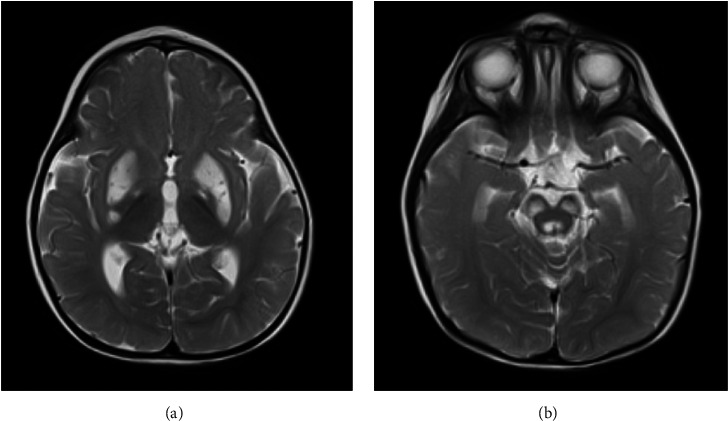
Brain MRI in the patient at the age of 51 weeks. (a) Axial, T2-weighted images showing bilateral high signal intensity in the lentiform nuclei, putamen, and globus pallidus. (b) Axial, T2-weighted images showing bilateral high signal intensity in the cerebral peduncles and midbrain.
